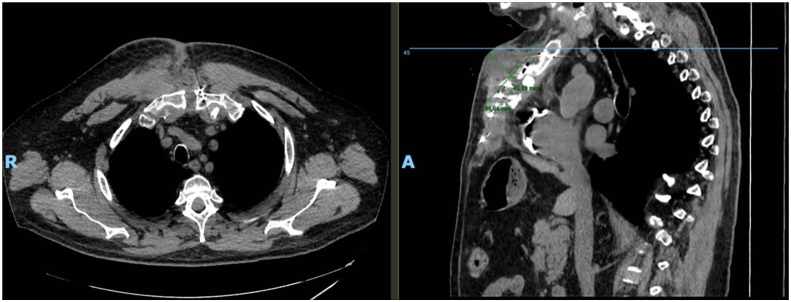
Axial and sagittal computed tomographic views of the chest that show a right anterior chest wall collection. (A, anterior; R, right.)
